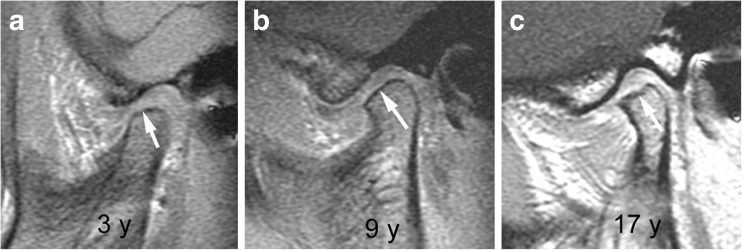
Moderate condylar flattening (grade 2). a-c. Sagittal oblique T1-weighted gradient echo images (TR/TE 300/4.2 ms, flip angle 80°, without fat saturation) from different children obtained at 3 years (a), 9 years (b) and 17 years (c) show flattening involving the entire surface of the condyle with preserved anterior angulation (arrows)
